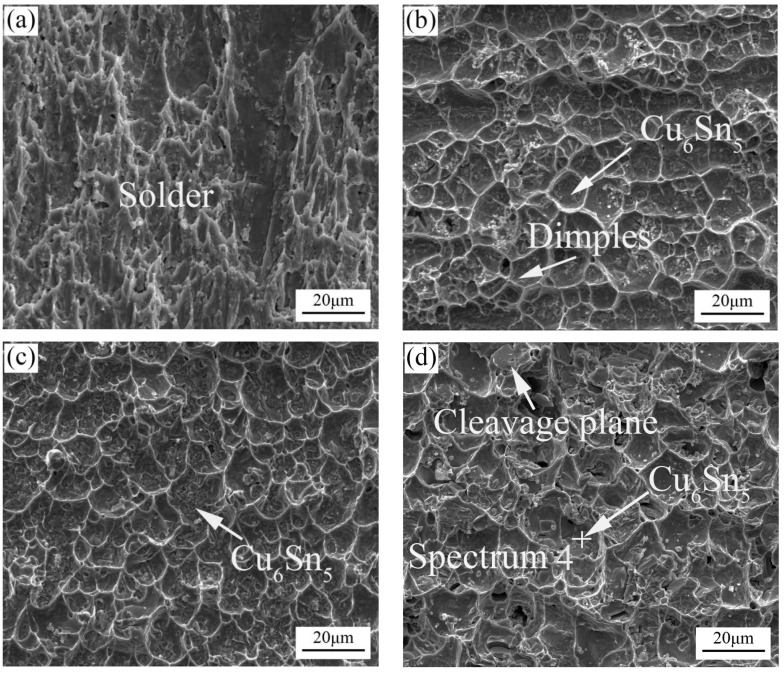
Top views of the shear fracture surfaces for the solder joints at 800-ms ultrasonic bonding times for different aging time: (a) 24 h; (b) 120 h; (c) 240 h and (d) 360 h.
